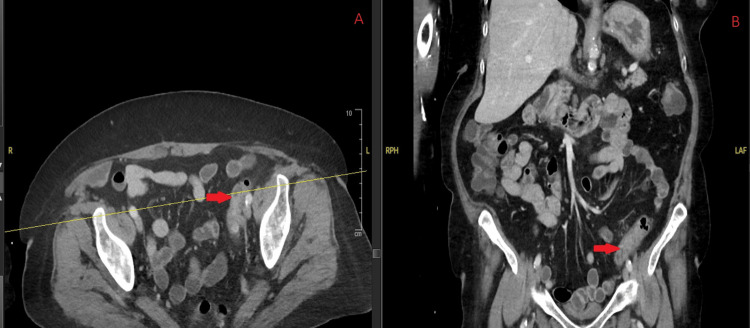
Contrast-enhanced CT of the abdomen obtained during readmission showing mild pericolic edema in the distal descending and proximal sigmoid colon consistent with uncomplicated diverticulitis (arrows). (A) Axial view. (B) Coronal view. CT: computed tomography
